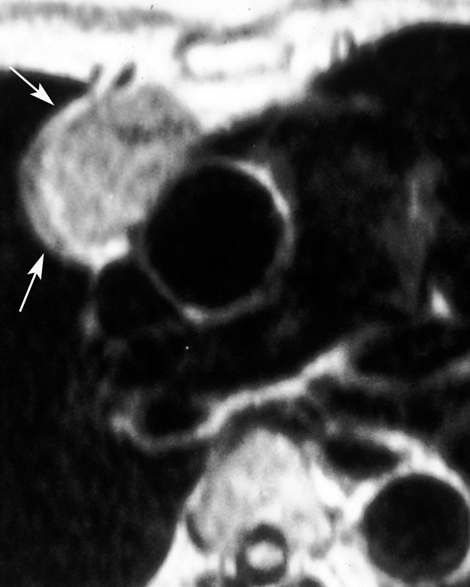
MRI of the chest revealed an anterior mediastinal mass consistent with a thymic tumor.
